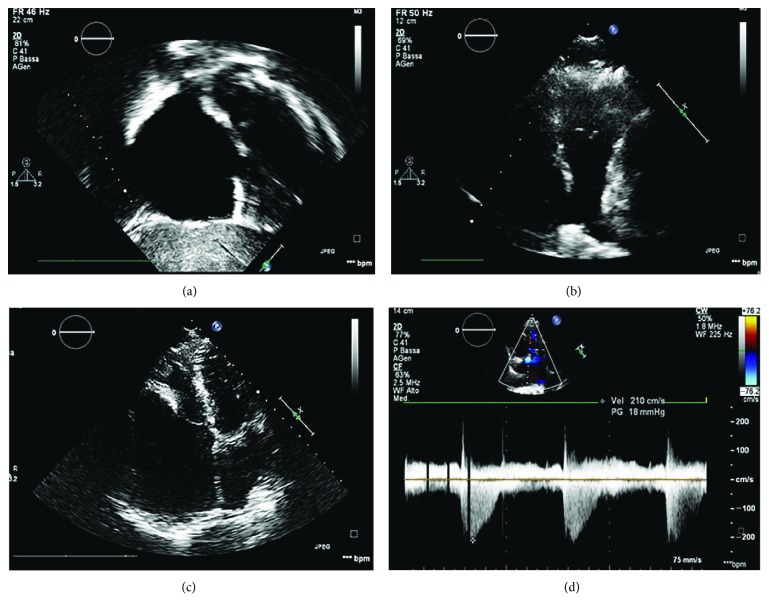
(a) Subxiphoid long-axis view showing abnormal dilation of the right atrium and intact interatrial septum. (b) Modified parasternal short-axis view showing the regular diameter of the pulmonary trunk. (c) Apical 4-chamber view demonstrating the compression of the right ventricle from the right atrium. (d) CW Doppler of tricuspid regurgitation.
